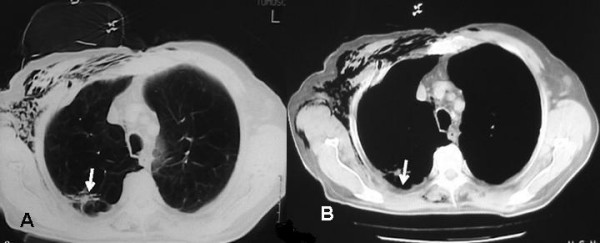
Preoperative chest CT scan. (A) Diffuse emphysematous changes of the right upper lobe, surgical subcutaneous emphysema and a scar-like lesion located in the periphery of the posterior segment of the right upper lobe (arrow). (B) Thickening of the parietal pleura in proximity to the scar-like lesion (arrow).
